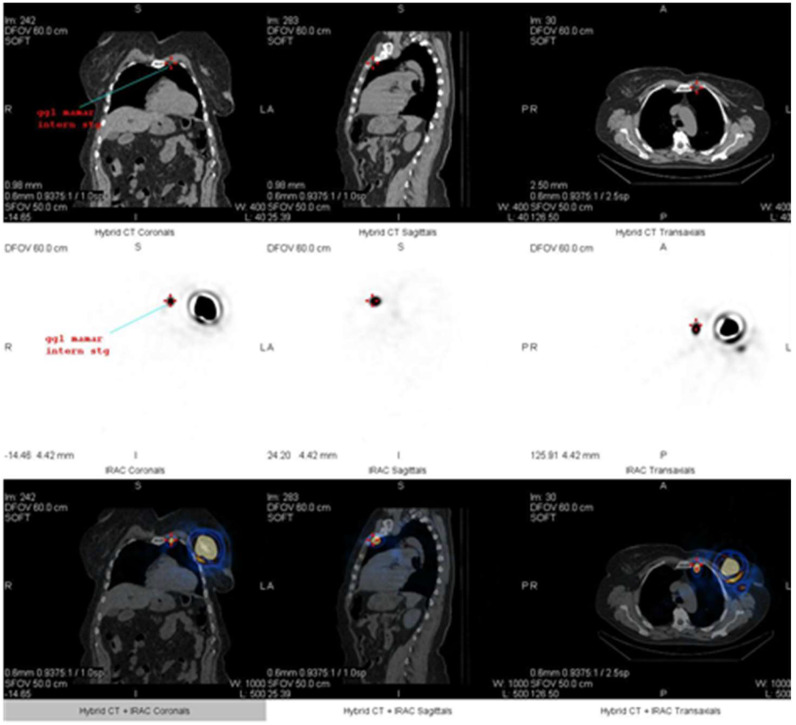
View of SPECT-CT with low dose of the SLN at the level of the left internal mammary chain: coronal, sagittal, and transverse images (CT, nuclear medicine, and fused series of images).
